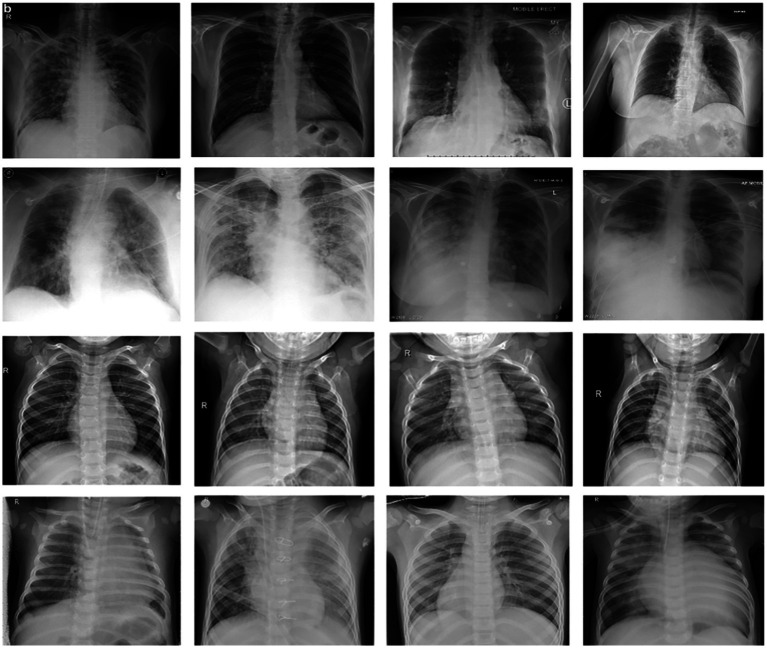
Sample of images from COVID-Xray-3k Dataset. First row corresponds to images with COVID-19. The second row corresponds to four sample images diagnosed with no COVID-19 infection from ChexPert, belonging to the no-finding category. The third and fourth row corresponds to images with eight samples belonging to all other subdomains in ChexPert.
